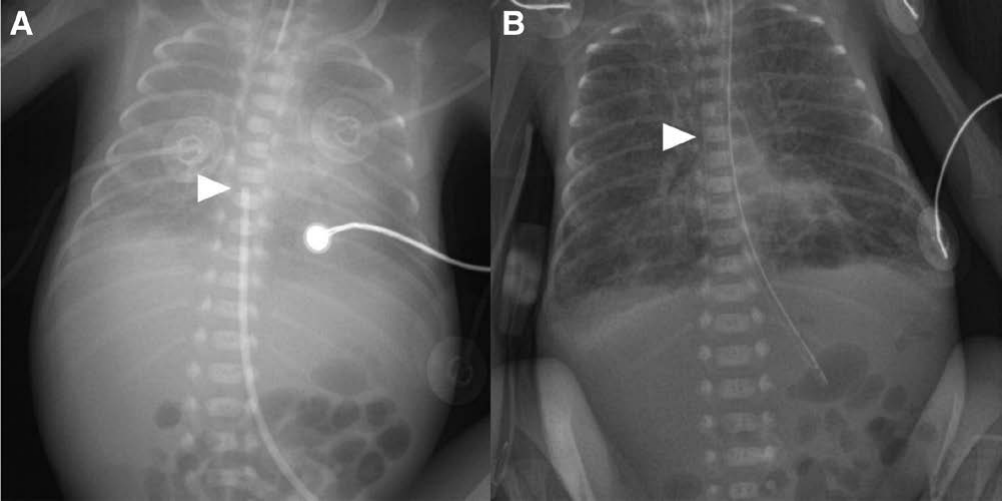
Chest radiography showed the position of initial central lines, case 1 patient. Umbilical venous catheterization was performed immediately after birth. The distal tip UVC was located at T8 level, indicated by a white arrow (A), PICC was inserted at 14th day of life. The position of distal tip of PICC was presumed at cavo-atrial junction (B). PICC = peripherally inserted central catheter, UVC = umbilical venous catheter.
